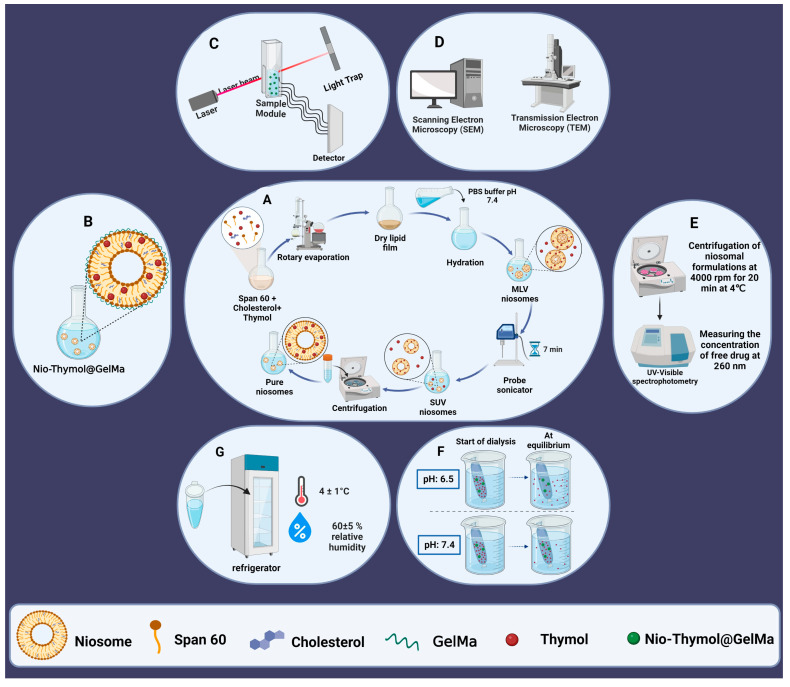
Fabrication and characterization of the niosomal formulations. (A) Preparation of niosomes using thin-layer hydration method, MLV: multilamellar vesicles, SUV: small uni-lamellar vesicles; (B) functionalizing niosomes with GelMa; (C) size determination; (D) morphology study; (E) entrapment efficacy study; (F) in vitro drug release study; and (G) stability study.
